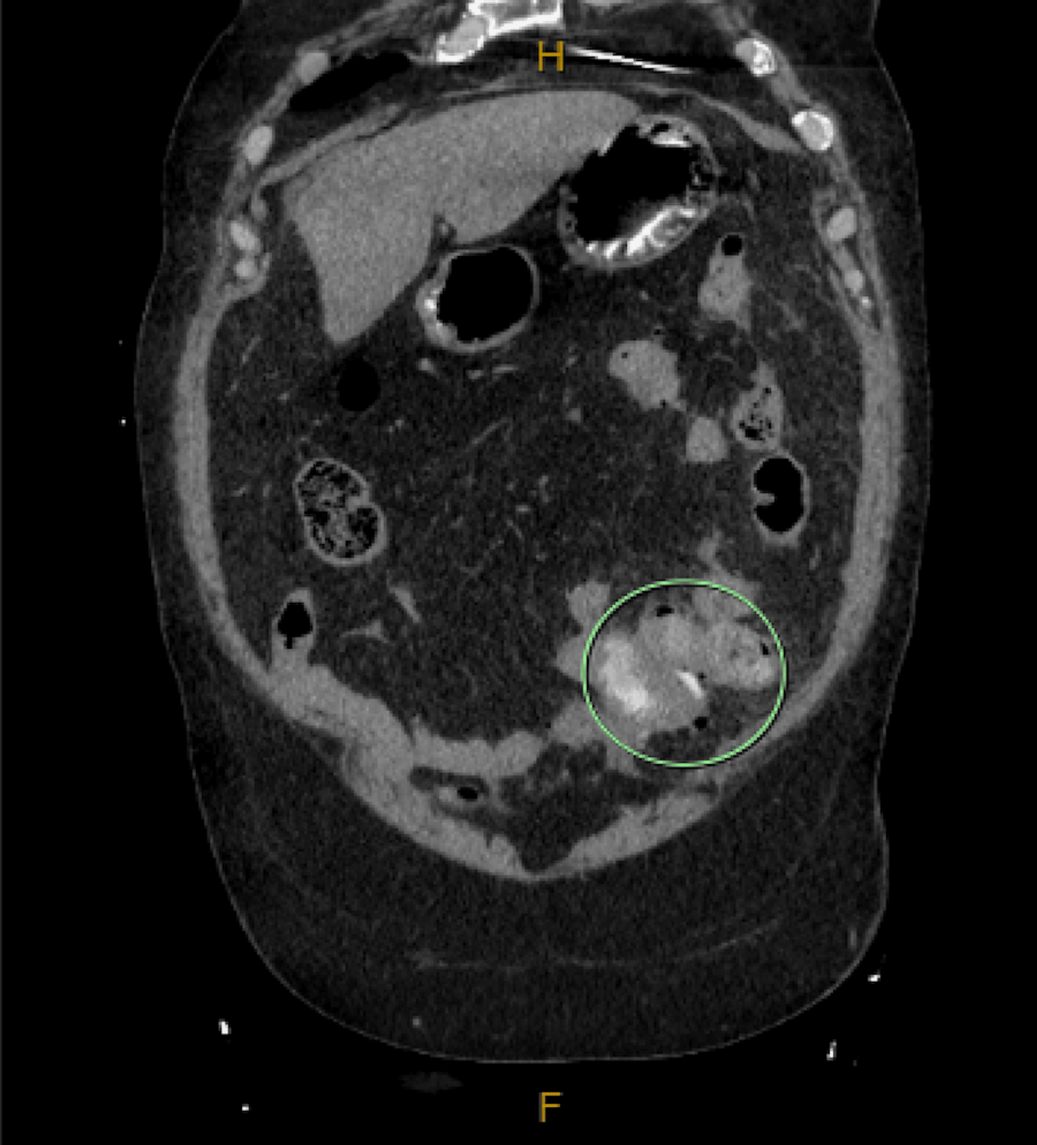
Coronal computed tomography (CT) image of the abdomen with oral and intravenous contrast showing mesenteric inflammation and intraperitoneal free air consistent with perforated small bowel diverticulitis
